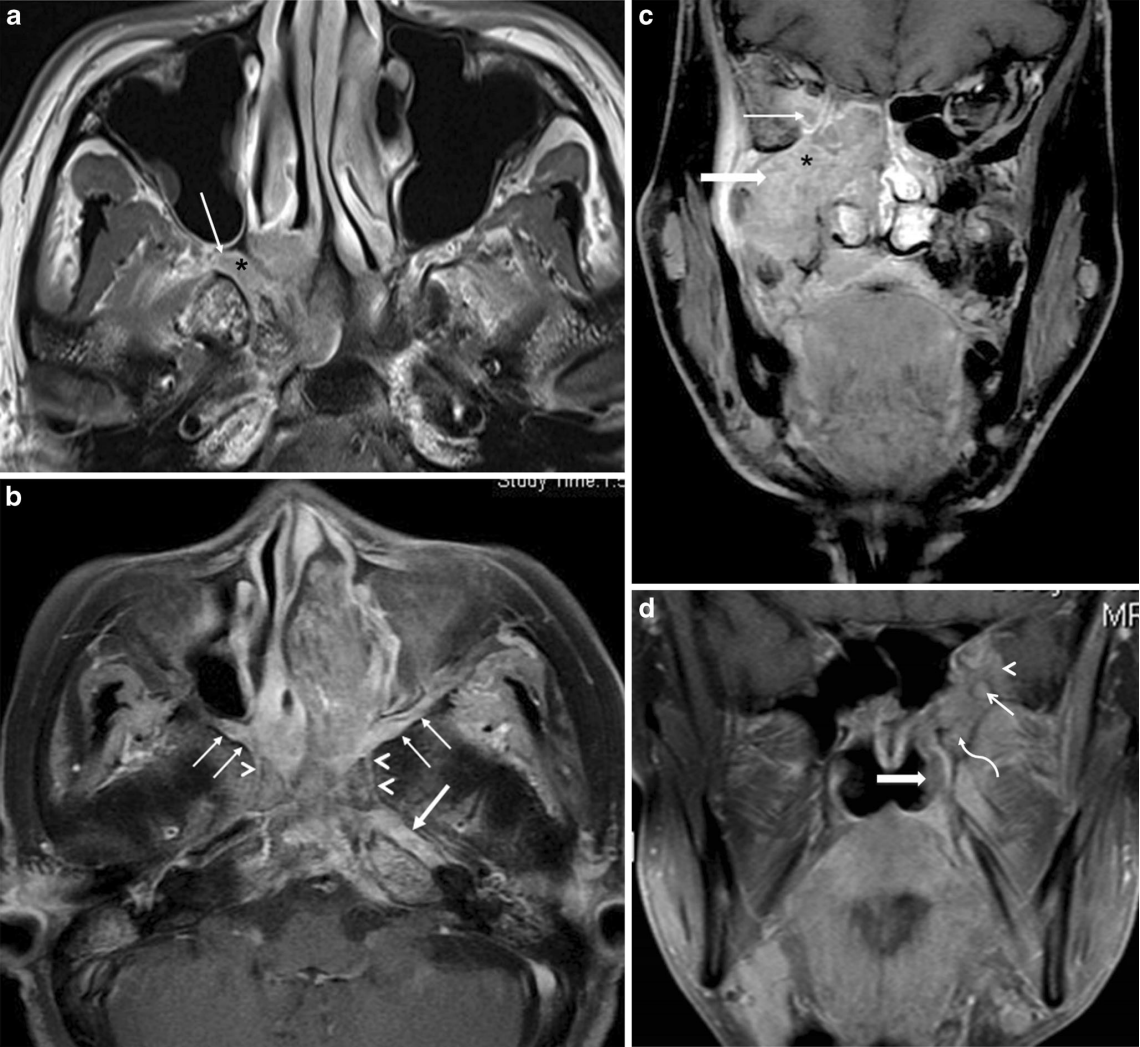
a NPC in a 50-year-old male patient. Axial contrast-enhanced T1W MR image shows an enhancing mass in the right side of the nasopharynx with direct extension into the medial half of the right PPF (*arrow*) via the right SPF (*asterisk*). b NPC in a 46-year-old male patient. Axial contrast-enhanced, FS T1W MR image shows an enhancing mass in the nasopharynx extending into the left nasal cavity and showing bilateral involvement of the PPFs (*thin arrows*). From the PPFs, there is retrograde PNS via bilateral Vidian nerves (*arrowheads*) with extension up to the left foramen lacerum (*thick arrow*). c Extensive stage 4 NPC in a 60-year-old male patient. Coronal contrast-enhanced FS T1W MR image shows extension of the tumour into the right ITF (*thick arrow*) and into the right orbit (*thin arrow*) via the PPF (*asterisk*). d Recurrent NPC in a 55-year-old female patient. Coronal contrast-enhanced FS T1W MR image shows an enhancing soft-tissue mass in the left side of the nasopharynx (*thick arrow*), with extension into the left PPF (*curved arrow*), leading to PNS along the left foramen rotundum (*thin straight arrow*). There is also involvement of the left middle cranial fossa (*arrowhead*)
